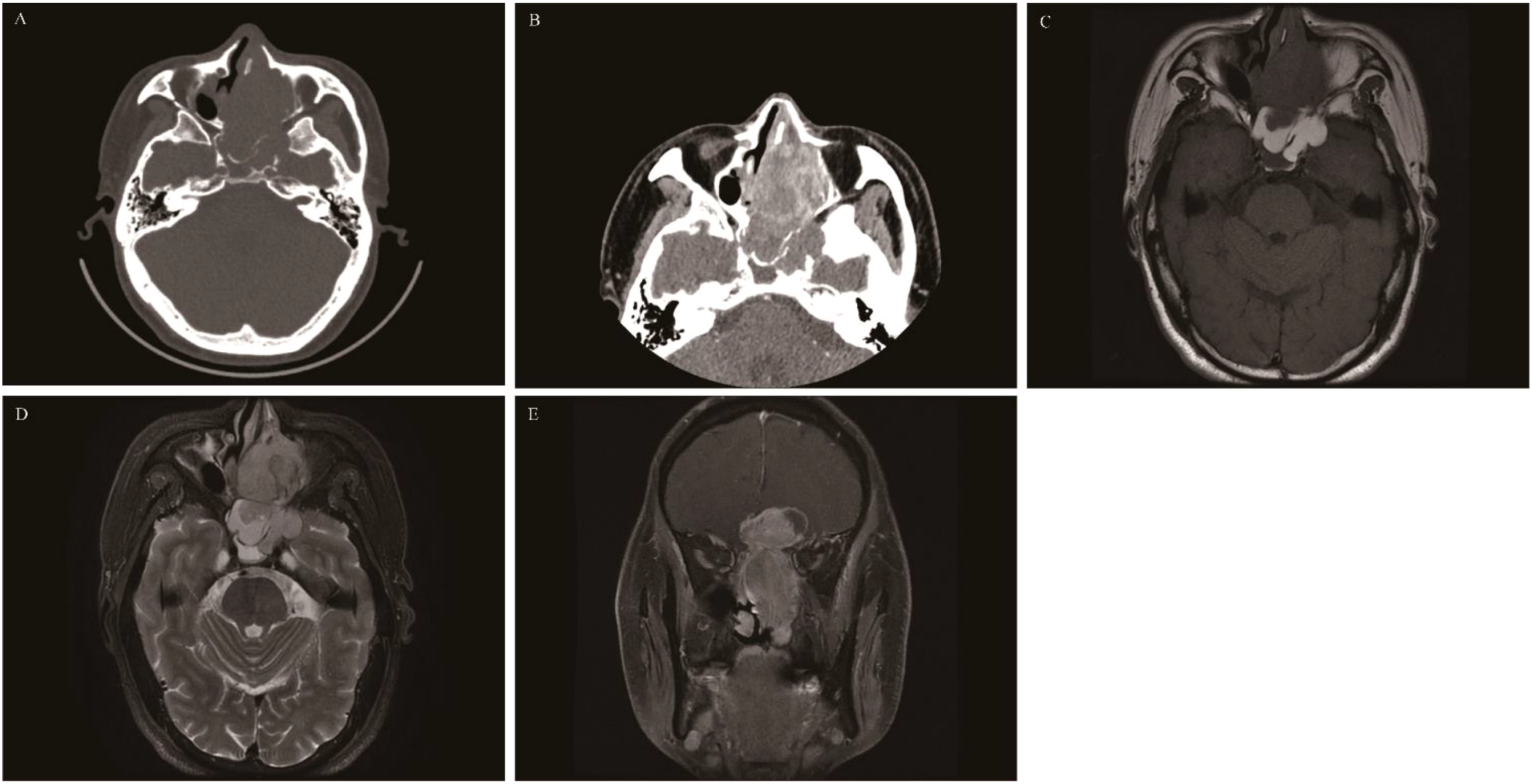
Sinonasal teratocarcinosarcoma: CT revealed an irregular soft tissue mass in the left ethmoid sinus with significant bone destruction and heterogeneous enhancement (A, B). On T1WI the mass appeared isointense (C), while on T2WI, it is slightly hyperintense (D). The coronal contrast-enhanced image depicted a “dumbbell-shaped” mass with pronounced enhancement (E), this presentation is often misdiagnosed as olfactory neuroblastoma.
